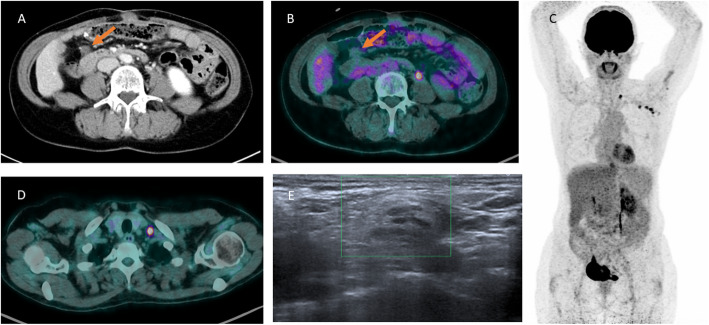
CT scan showed a peritoneal nodule (arrow in a) with no increased uptake on fused ^18^F-FDG PET/CT images (arrow in b). MIP (c) and fused ^18^F-FDG PET/CT (d) showed with increased uptake on the left axillary lymph nodes up to the left supraclavicular area. Echography (e) showed a normal supraclavicular lymph node
